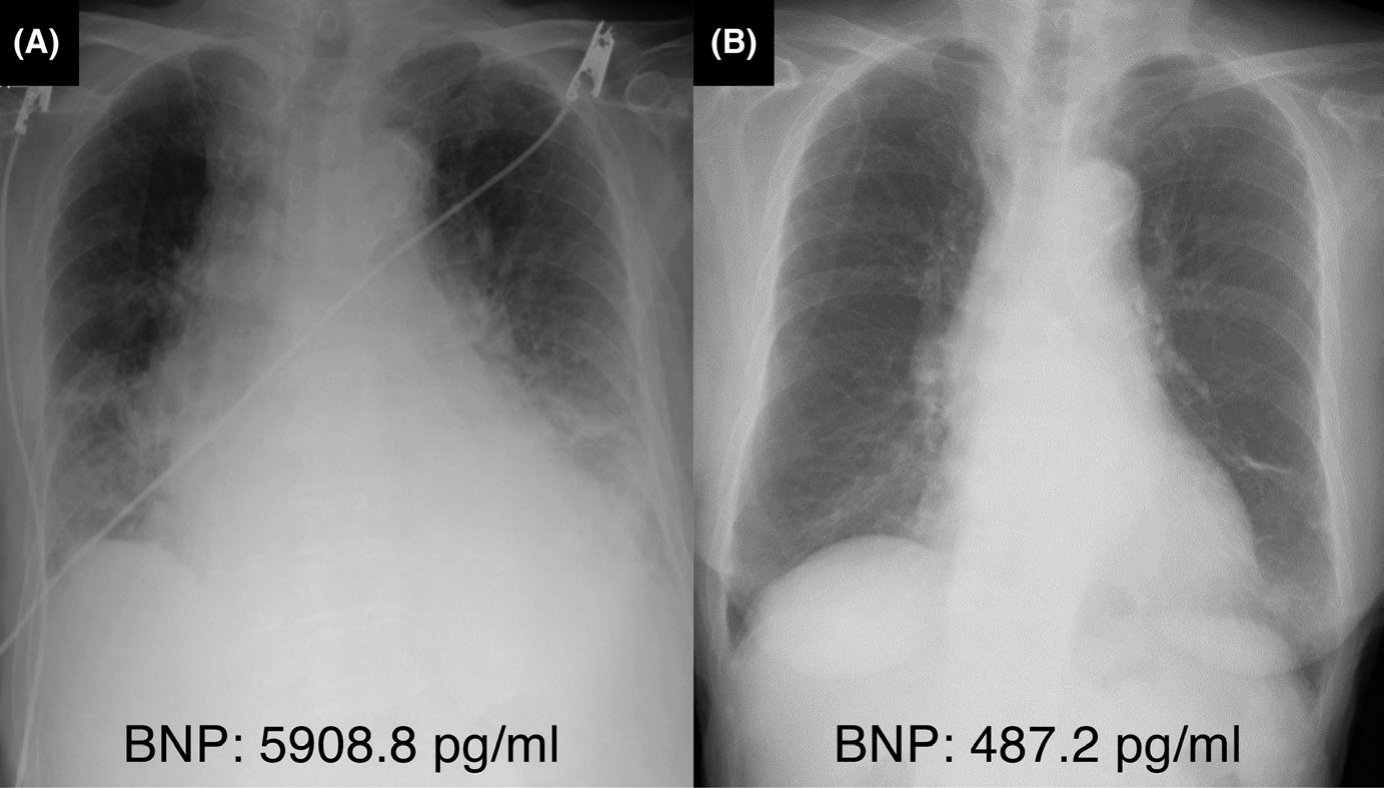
Chest radiographs and the brain natriuretic peptide (BNP) levels before and after retrograde balloon aortic valvuloplasty (BAV). (A) Preprocedural chest radiograph at day 1 showing severe pulmonary congestion with a butterfly shadow. (B) Postprocedural chest radiograph at day 8 showing resolution of pulmonary edema.
